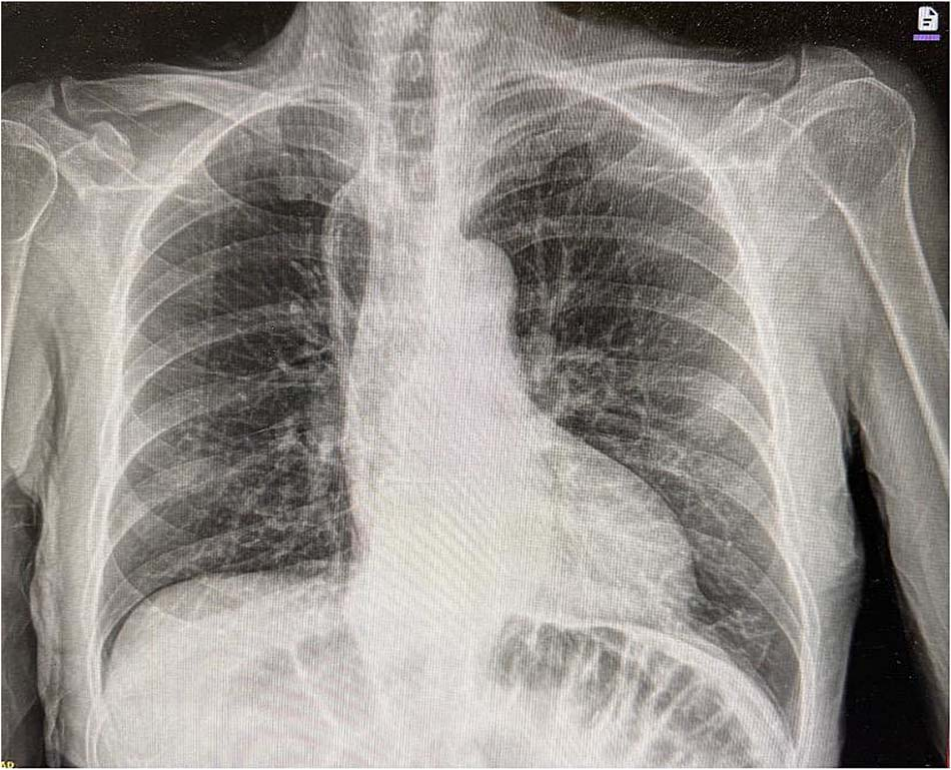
Postoperative chest X-ray imaging.
